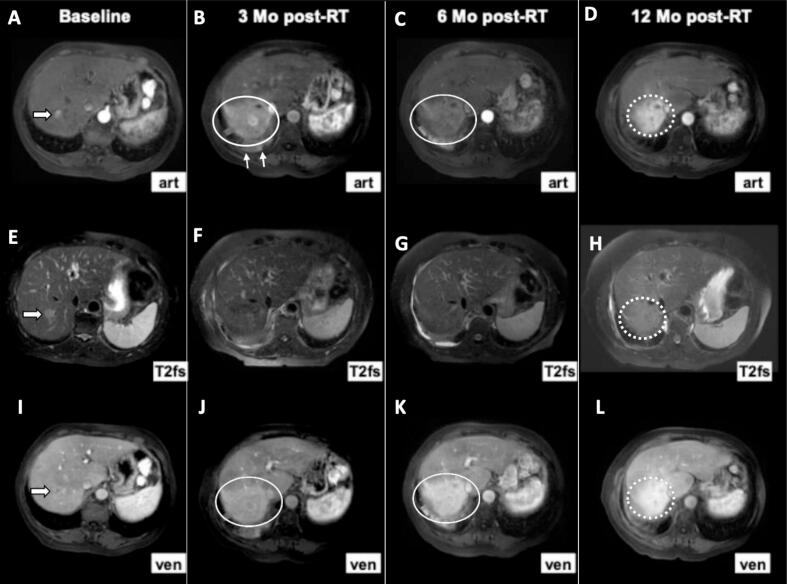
Multiparametric MRI appearance of HCC and surrounding liver parenchyma after SBRT. Axial T1-weighted contrast-enhanced arterial phase (A–D), axial T2-weighted fat-suppressed (E–H), and axial T1-weighted contrast-enhanced portal venous phase (I–L) images. The baseline examination shows a T2-weighted hyperintense lesion in segment VII with non-rim arterial hyperenhancement (APHE) and portal venous washout (arrows in A, E, I), typical for HCC. After SBRT, serial MRI demonstrates temporal and spatial changes that help differentiate treatment response from local tumor progression and benign radiation-induced effects. Within the treated lesion, early post-treatment findings include persistent APHE (B) and washout (J), followed by gradual conversion to delayed enhancement or non-enhancement (D, K, L), with increasing T1-weighted and decreasing T2-weighted signal (F—H). In contrast, local progression would show persistent or increasing, nodular APHE with washout and increased T2-weighted signal. A focal liver reaction surrounding the lesion is frequently seen, typically manifesting as ring-like or perilesional arterial enhancement (B—D) and diffuse T2-weighted hyperintensity (F—H) eventually leading to volume loss and retraction of the surrounding parenchyma; these perilesional and parenchymal changes may persist for months and should not be mistaken for residual or recurrent tumor. Note also the adjacent radiation-induced lung atelectasis next to segment VII (arrow in B). Abbreviations: art = arterial phase, ven = portal venous phase, T2fs = T2-weighted fat-suppressed.
